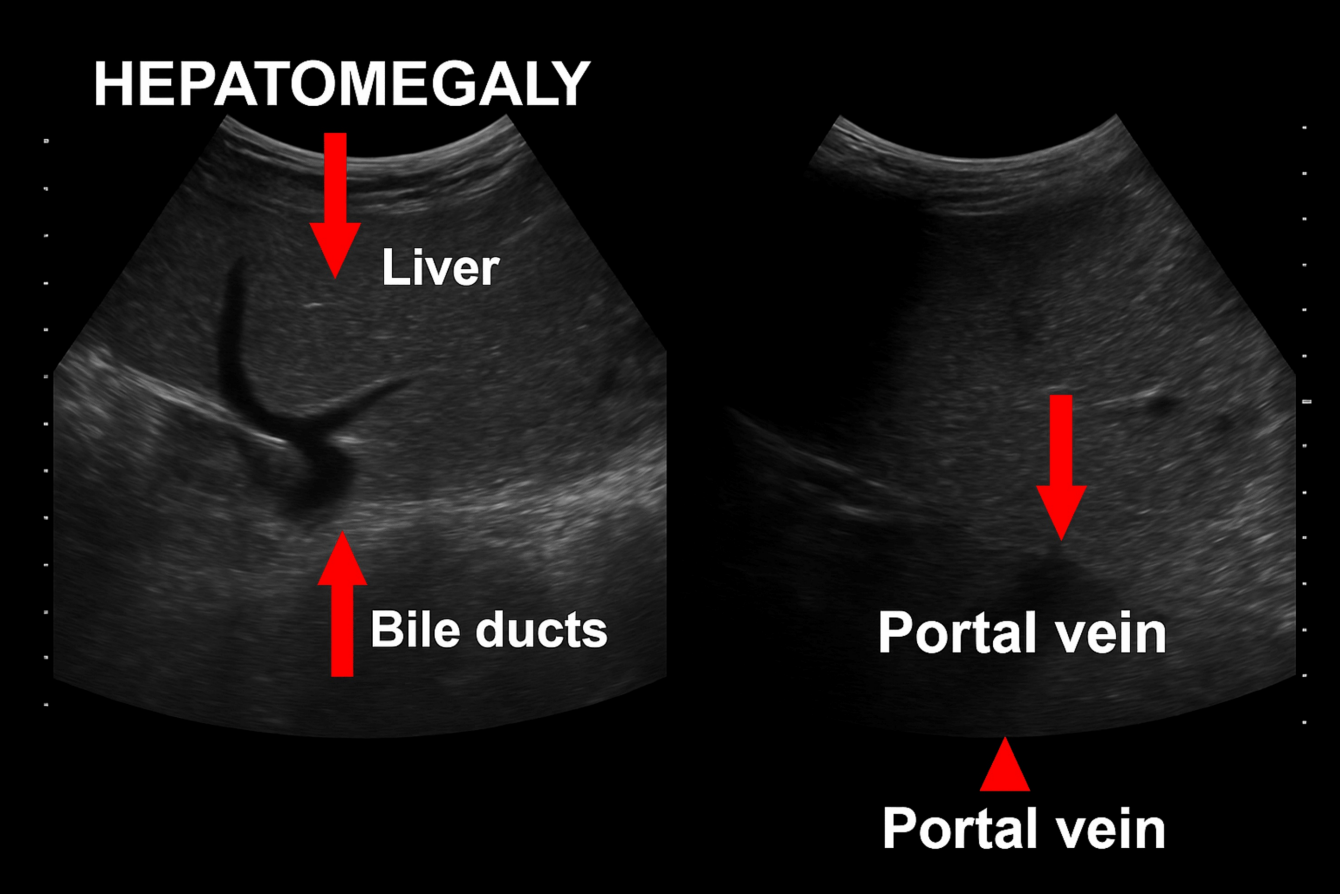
Ultrasonography image of case 1
